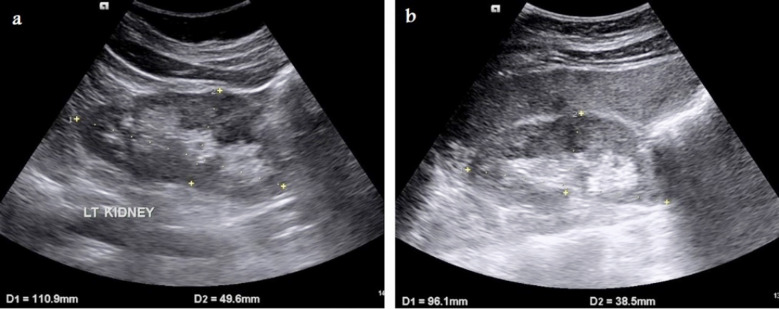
Selected gray-scale ultrasonography longitudinal sections of both kidneys in a 45-year-old female diabetic patient referred for follow up ultrasound imaging that show normal renal measurements (length and width) of a) left kidney, and b) right kidney.
